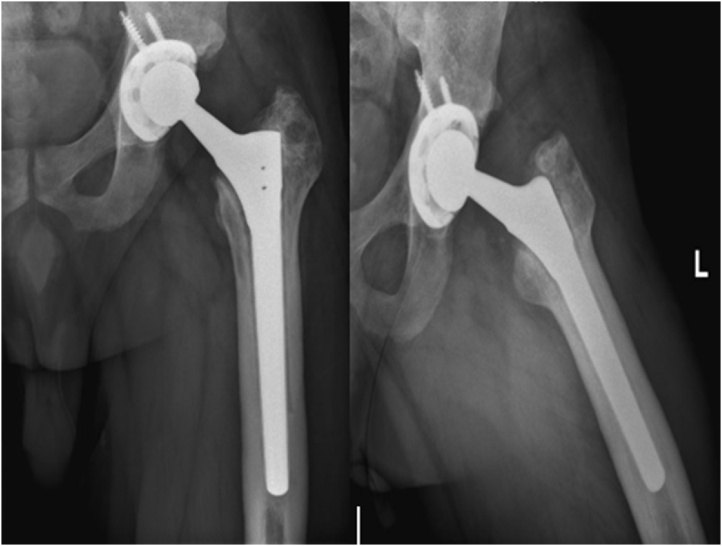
Latest anteroposterior and lateral X-rays of the left hip.
